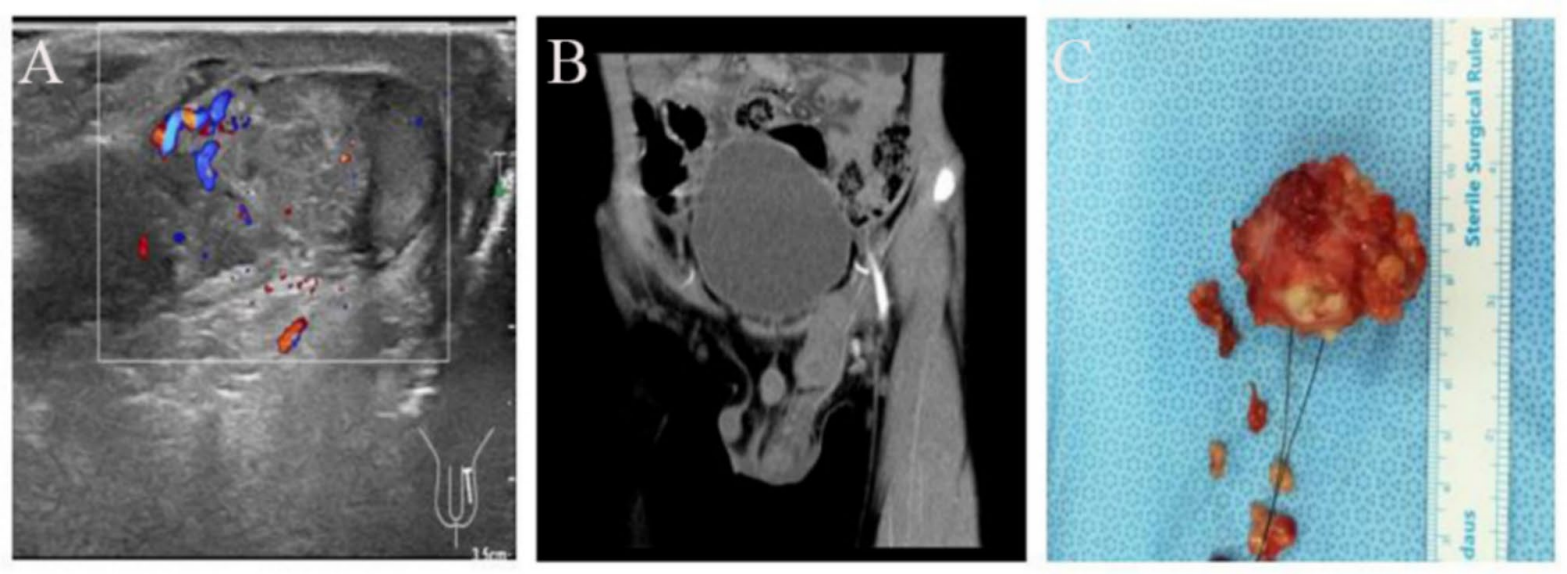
Patient (Case 9) misdiagnosed with a left inguinal hematoma. A: Ultrasound examination showed a mass with blood signals. B: Contrast-enhanced computed tomography scan. C: Surgically removed mass
